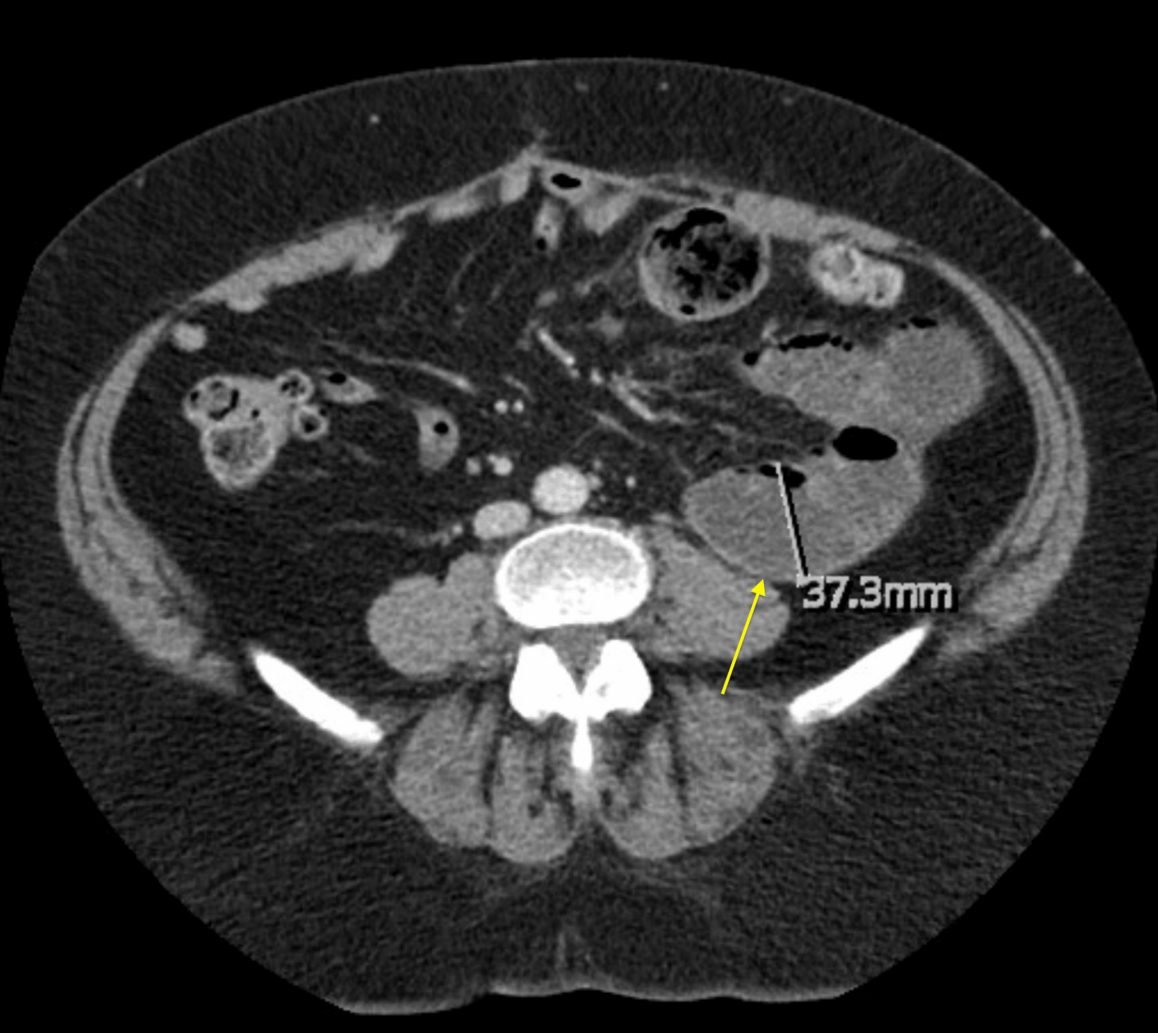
Axial CT scan shows prominent small bowel loops within the left upper and mid-abdomen measuring up to 3.7 cm (yellow arrow), consistent with SBO. CT: computed tomography; SBO: small bowel obstruction.
